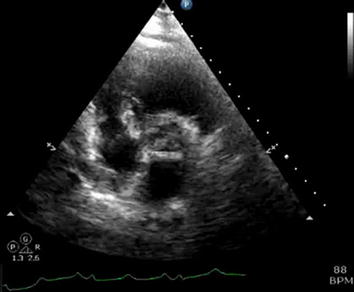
Parasternal short axis view of large mobile thrombus in the right atrium
